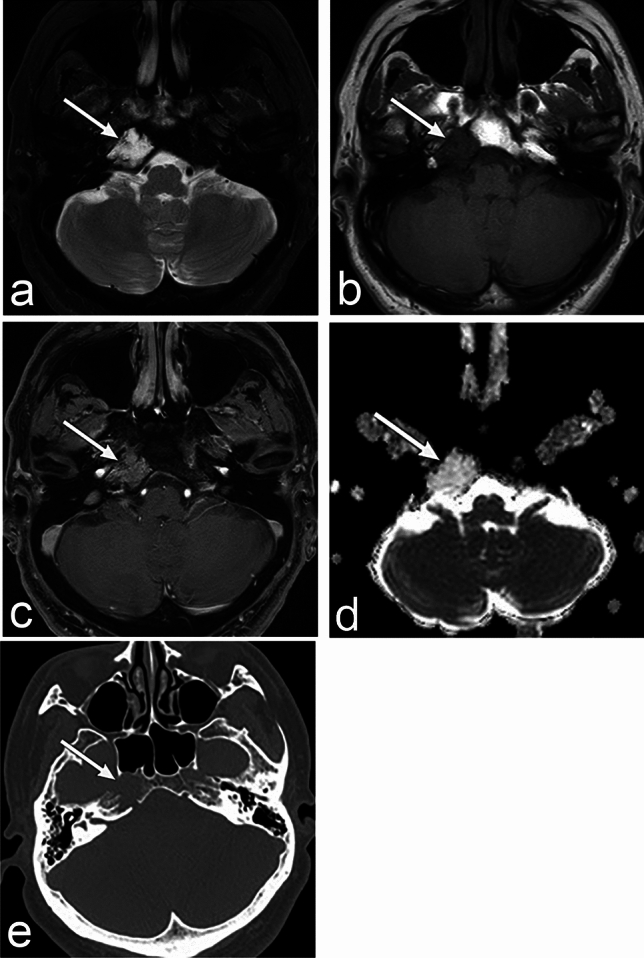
Chondrosarcoma of a man in his 50s with right diplopia. a–d MRI shows a well-defined mass (arrow) in the right temporo-occipital junction. The mass shows homogeneous high signal intensity on T2 weighted image (WI) and homogeneous low signal intensity on T1WI with heterogeneous moderate enhancement. The apparent diffusion coefficient value is as high as 1.89 × 10^–3^ mm^2^/s. e CT shows a mass (arrow) with the destruction of the clivus without calcification
